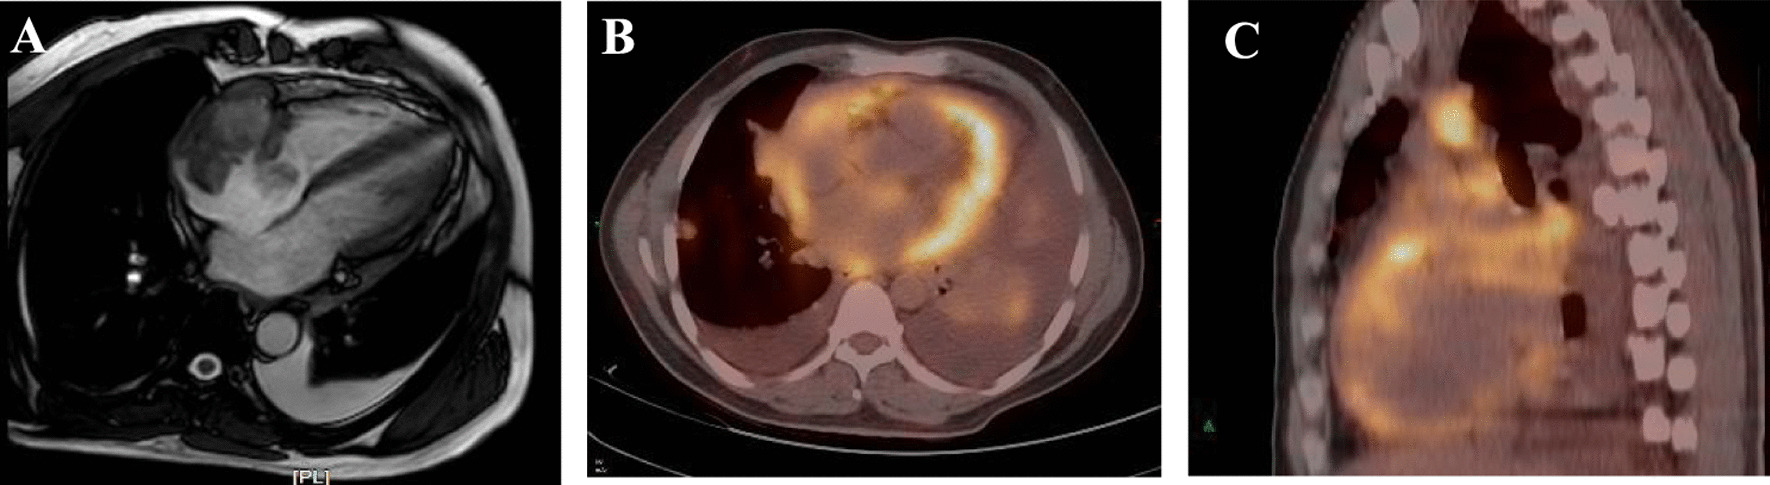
CMR shows the right atrium soft tissue mass closed to the tricuspid orifice (a). PET/CT scan suggests intensive tracer uptake of the right atrial mass, pericardium, lung and mediastinal lymph nodes (b, c)
